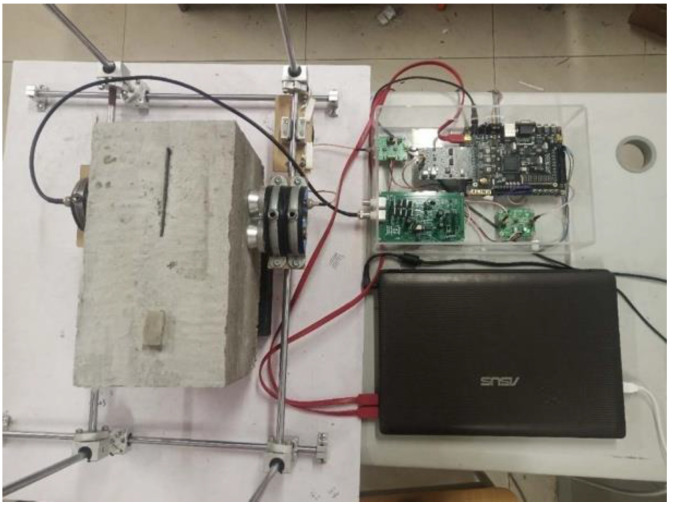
Ultrasonic detection hardware system.
